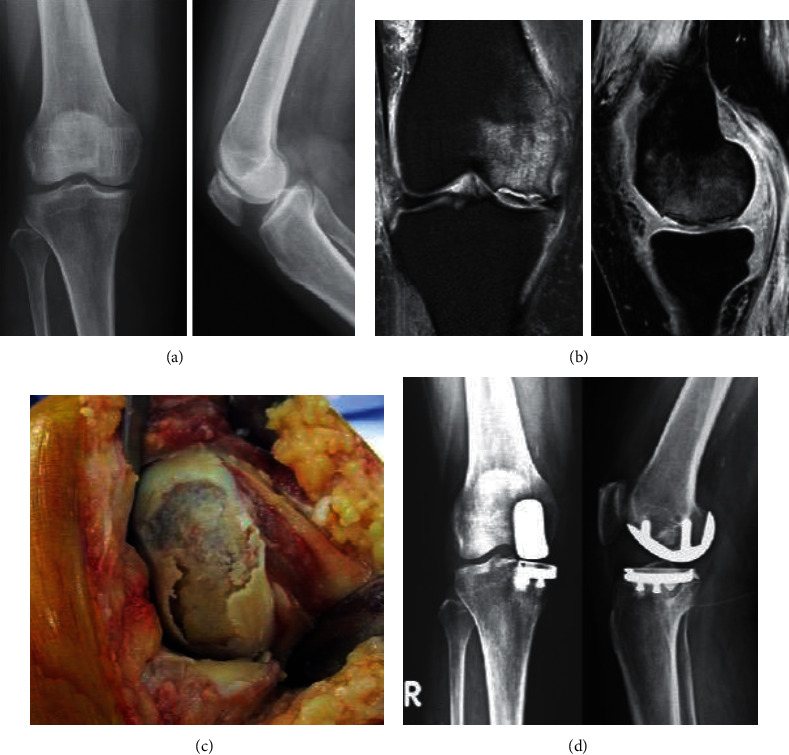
(a) Anteroposterior and lateral knee X-rays showing medial condyle flattening with joint space narrowing. (b) T2-weighted magnetic resonance images showing osteochondral fractures of the medial femoral condyle with marked marrow edema. (c) Clinical picture taken during surgery showing a massive osteochondral fracture in medial femoral condyle. (d) Knee X-rays showing reconstruction status postunicompartmental knee arthroplasty.
